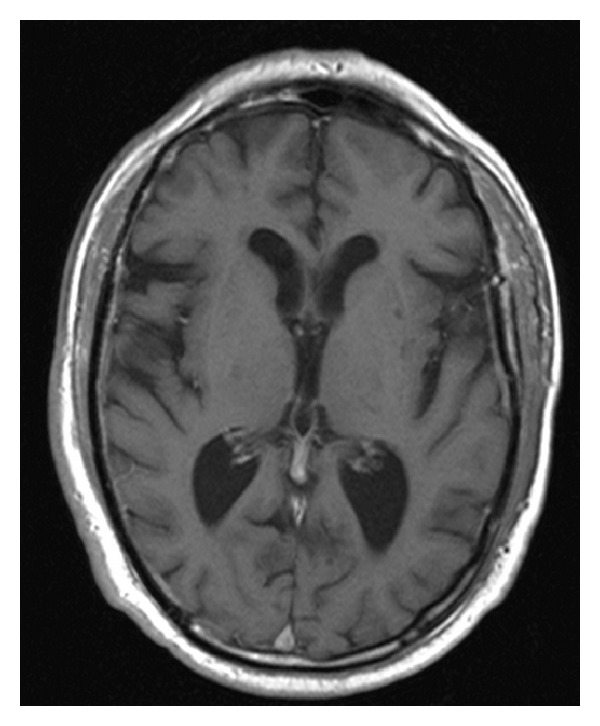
MRI T1—lacunar infarction in left putamen.
